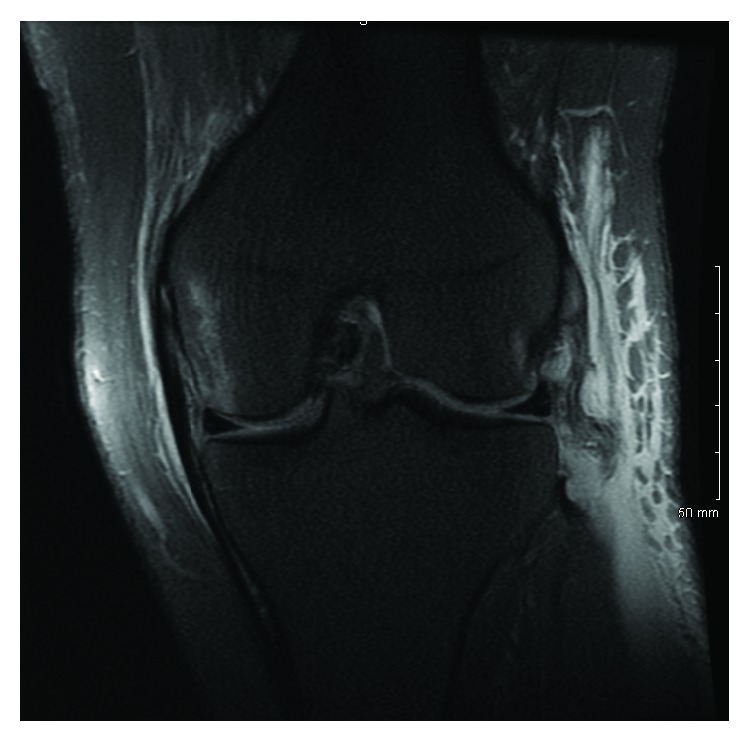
A T2 coronal MRI of the patient's left knee showing a grade I MCL injury with associated subchondral edema in the medial condyle.
